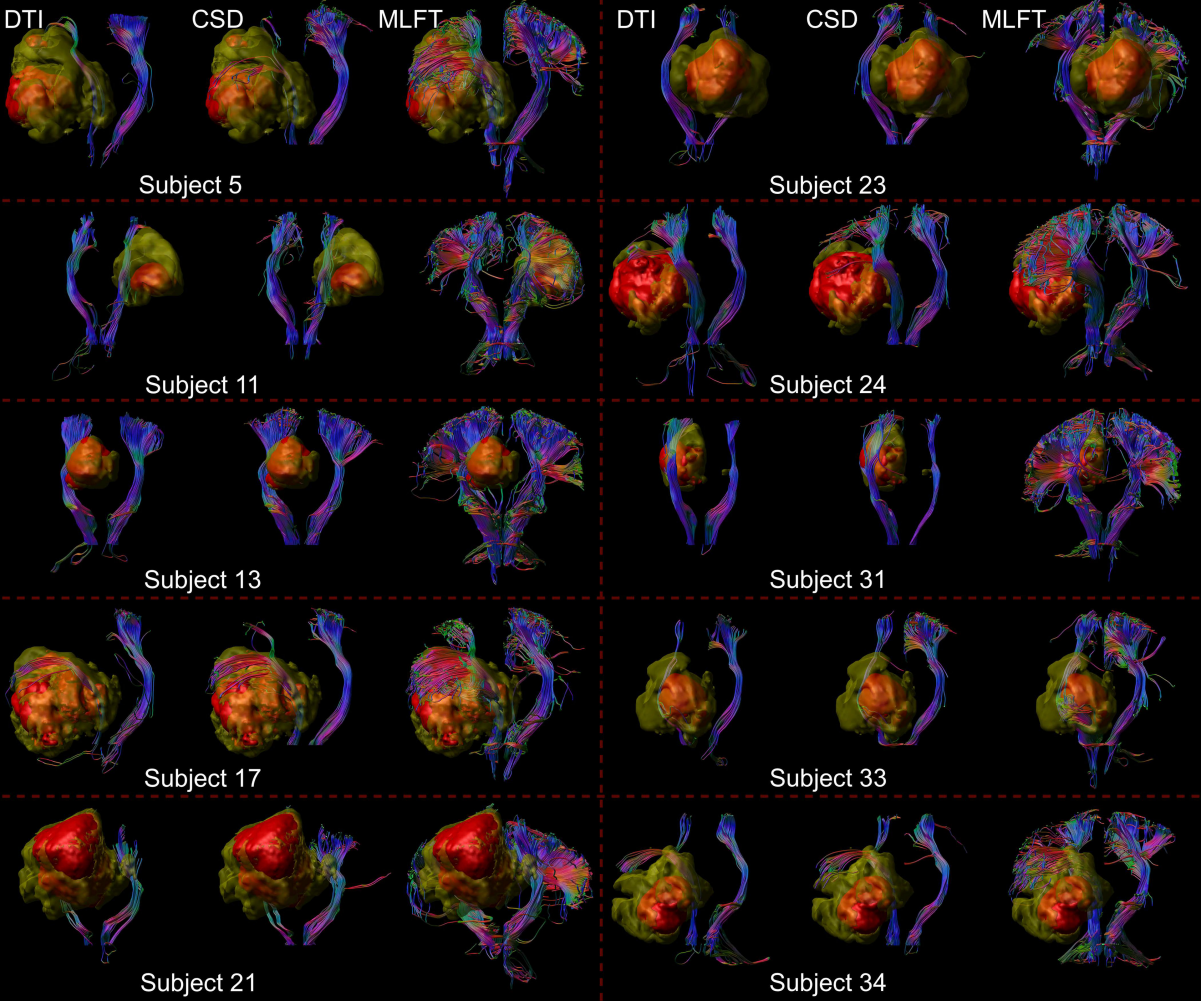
Comparison of reconstructions of the corticospinal tract (CST) depending on the algorithm chosen for tractography. This figure shows reconstructions of the CST within the tumor-affected and unaffected hemispheres in a subset of 10 patients from the cohort, using diffusion tensor imaging (DTI)-based tractography, constrained spherical deconvolution (CSD)-based tractography, and multi-level fiber tracking (MLFT). The tumor core is shown as a red volume, the hyperintense zone in fluid attenuated inversion recovery (FLAIR) sequences is shown as a yellow volume. While CSD-based tractography provides reconstructions comparable to DTI-based tractography, MLFT is able to improve depiction of the extent of the CST fanning of both tumor-affected and unaffected hemispheres.
